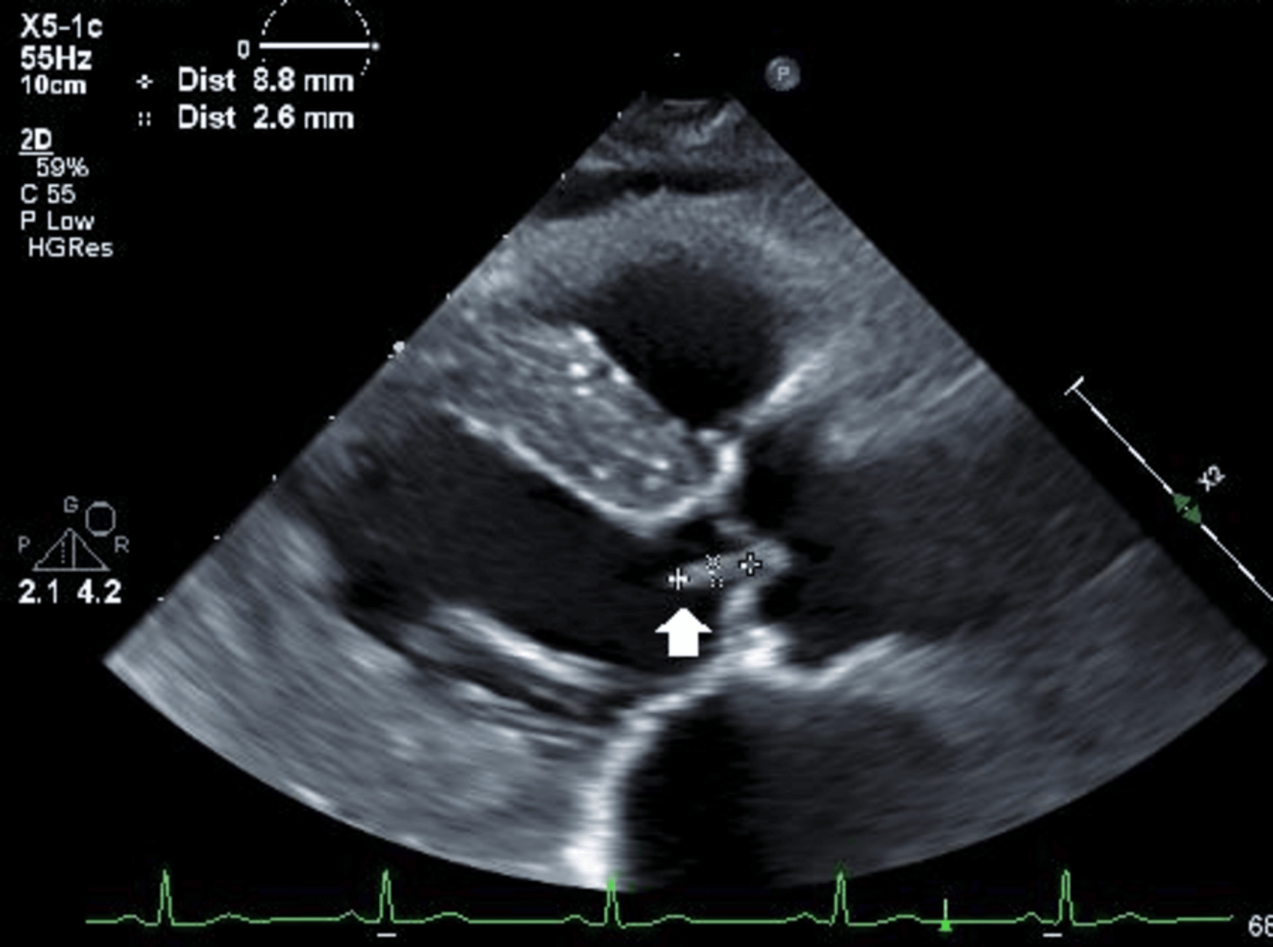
TTE image with parasternal long-axis view A new 9-mm mobile mass on the left coronary cusp of the aortic valve was revealed, suggesting a completely different lesion (arrow). TTE: transesophageal echocardiography.
